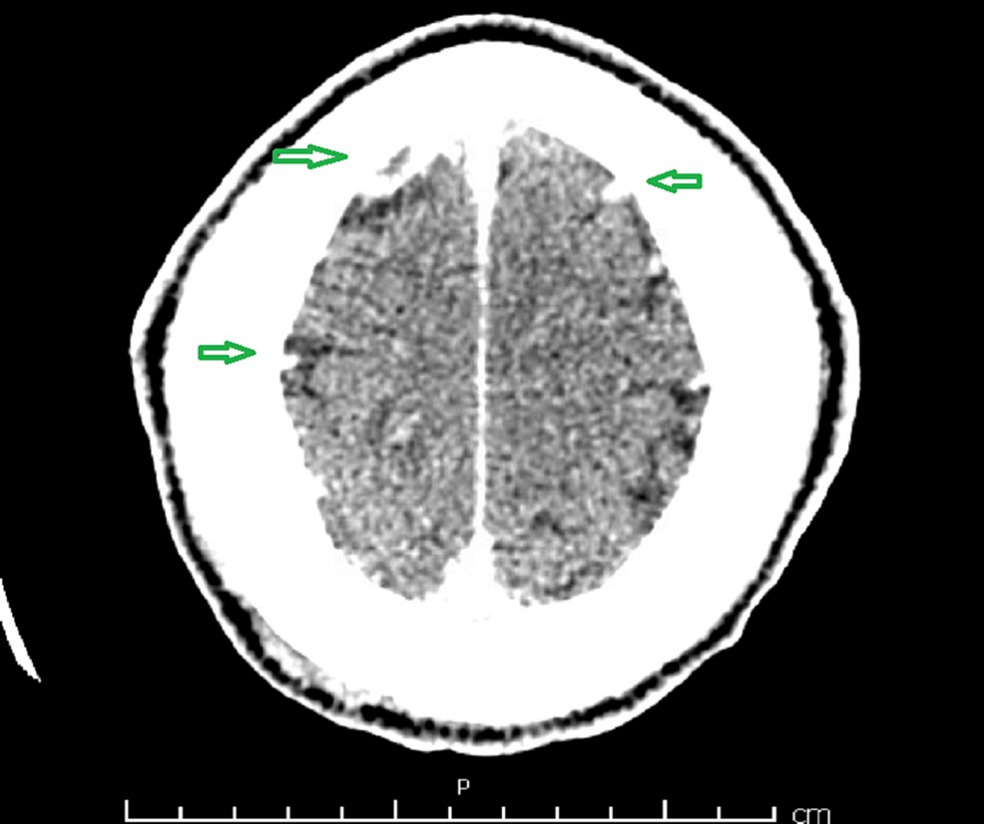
Computed tomography (CT) scan showing areas of cortical hypodensities with hyperdensities representing hemorrhagic stroke bilaterally as shown with green arrows
